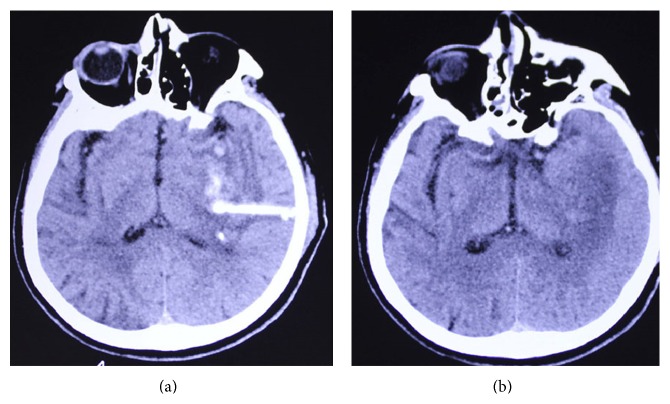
Cranial CT images of a patient before (a) and 6 months after BMSCs transplantation (b).
